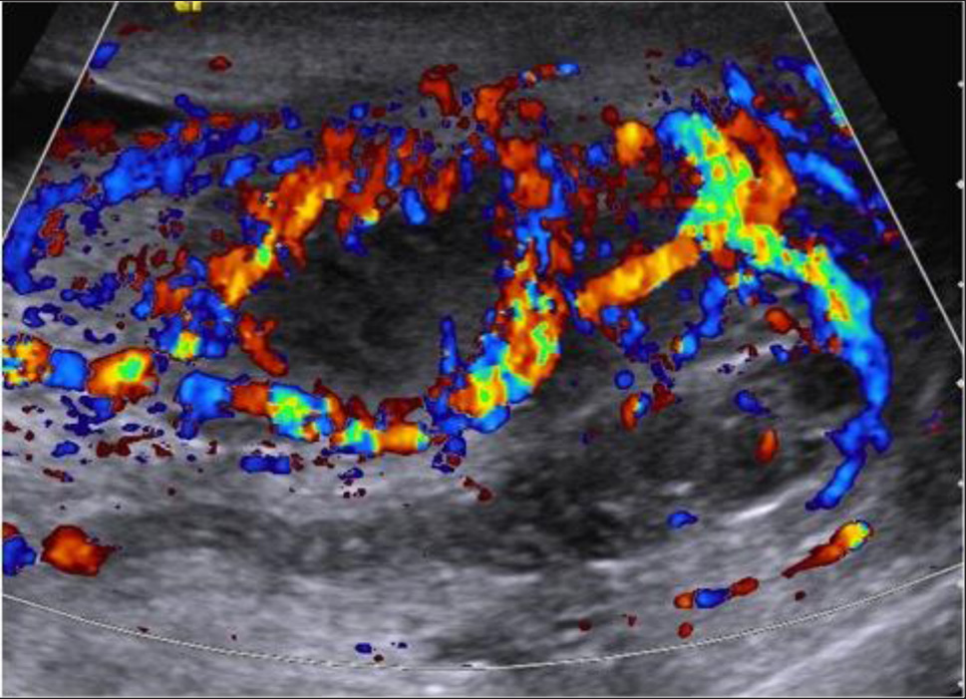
Longitudinal color Doppler image shows hypoechoic abscess of the hilum extending to the epididymis with increased flow signals in peripheral portion.
